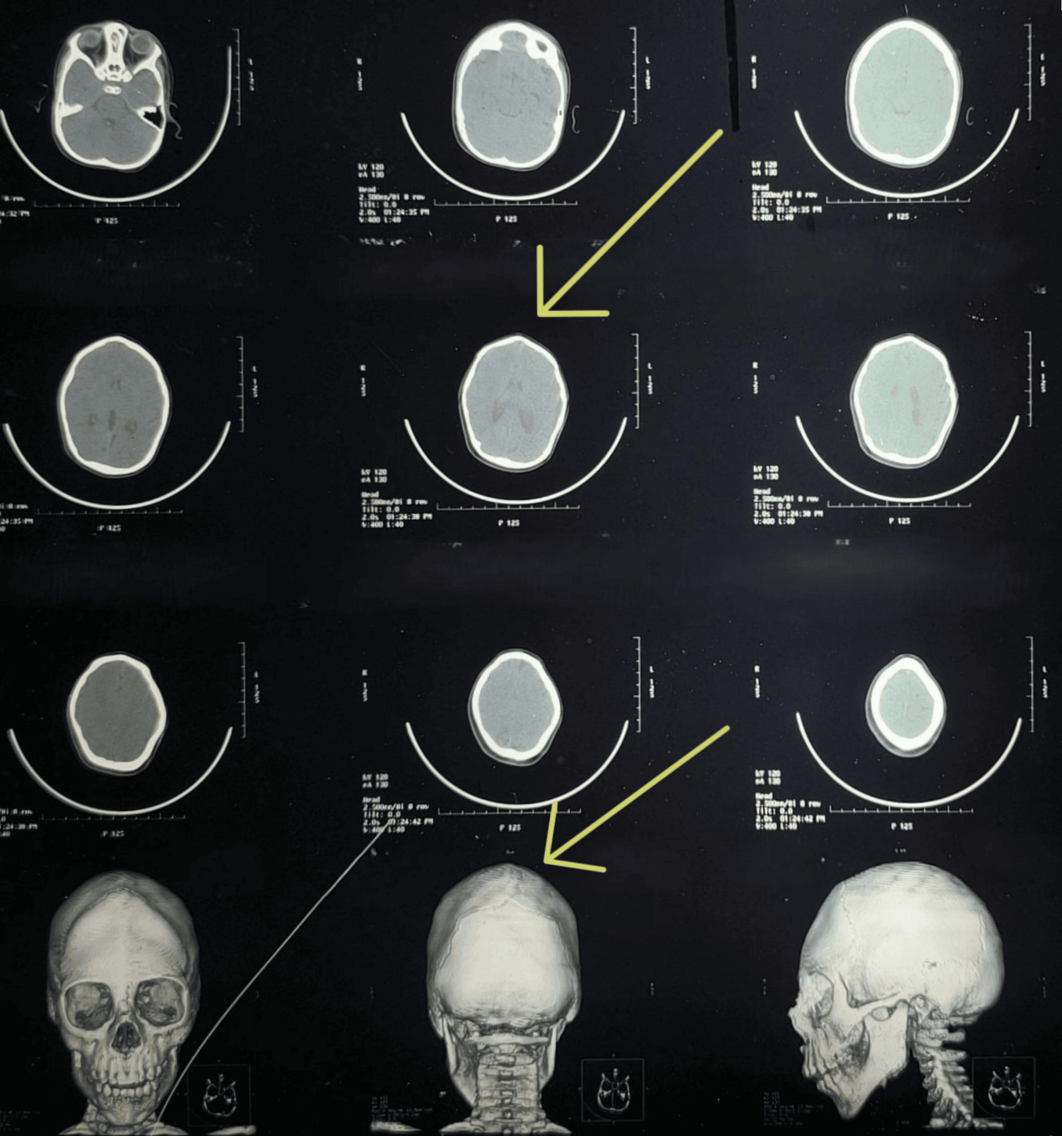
CT scan of the brain demonstrating premature fusion of the sagittal suture
